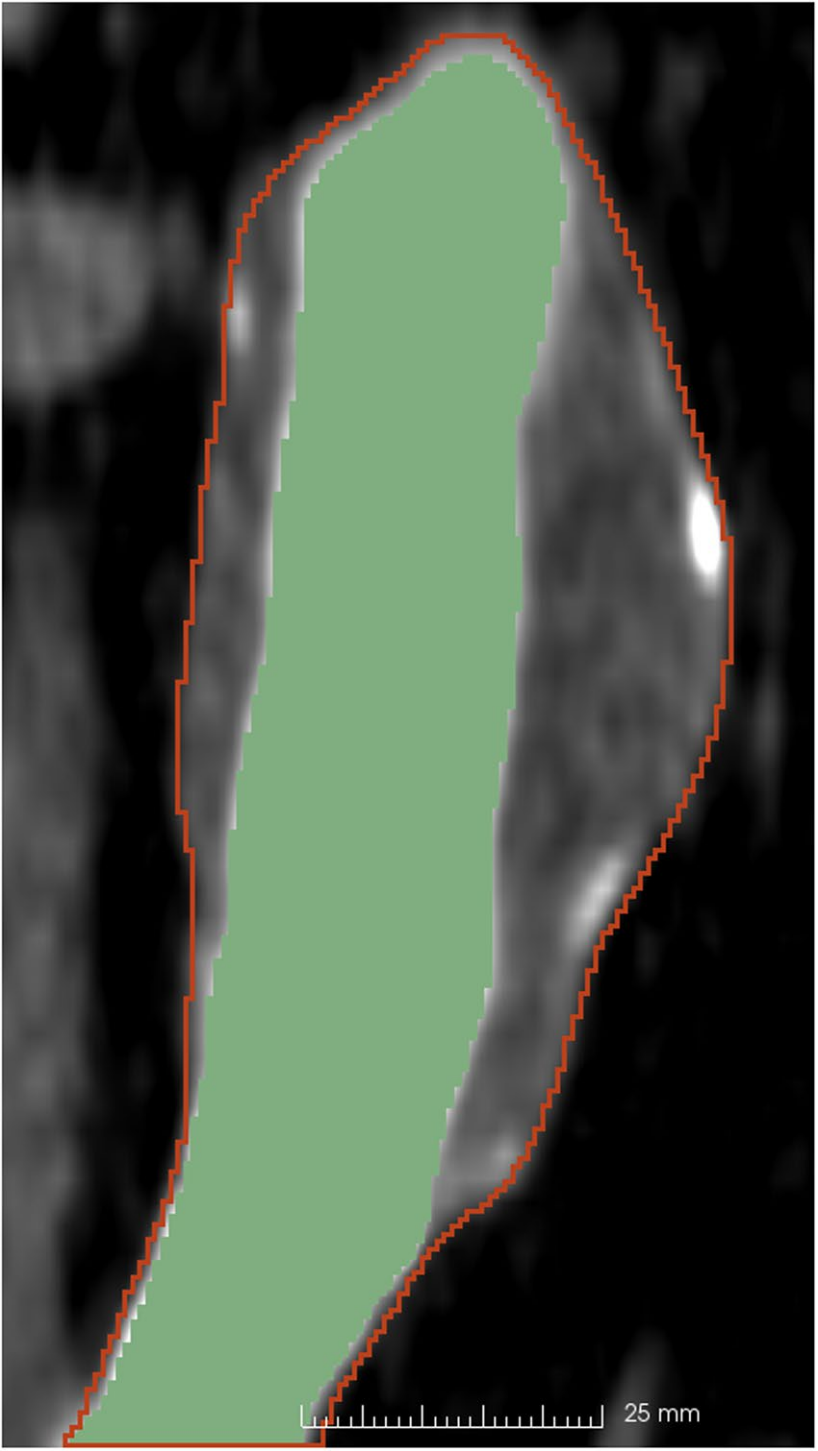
Example segmentation of an AAA from CT (one CT slice in the coronal view). The contour of the AAA segmentation is shown in red and the lumen segmentation is in green. The region of interest has been extracted from an abdominal CT with a resolution of 0.625 mm × 0.625 mm × 2 mm and segmented using the FastGrowCut algorithm for the AAA and intensity thresholding for lumen (with manual seed creation and segmentation corrections).
